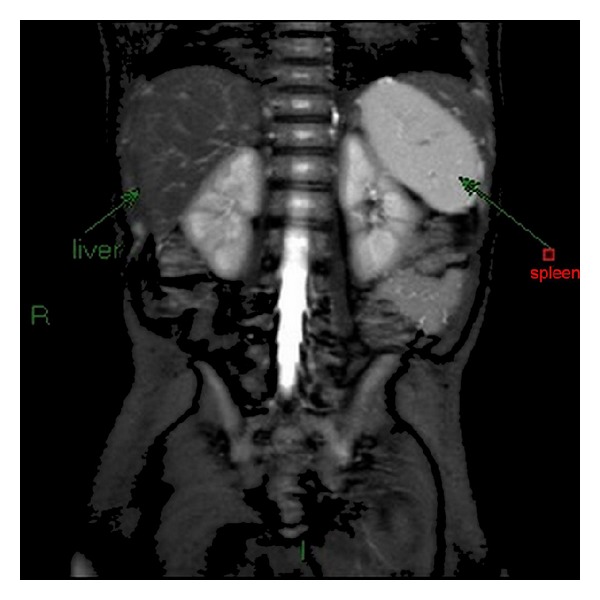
Follow-up abdominal MRI, coronal view, at 8 months showing normal intensity of liver parenchyma on T2WI with fat saturation protocol.
